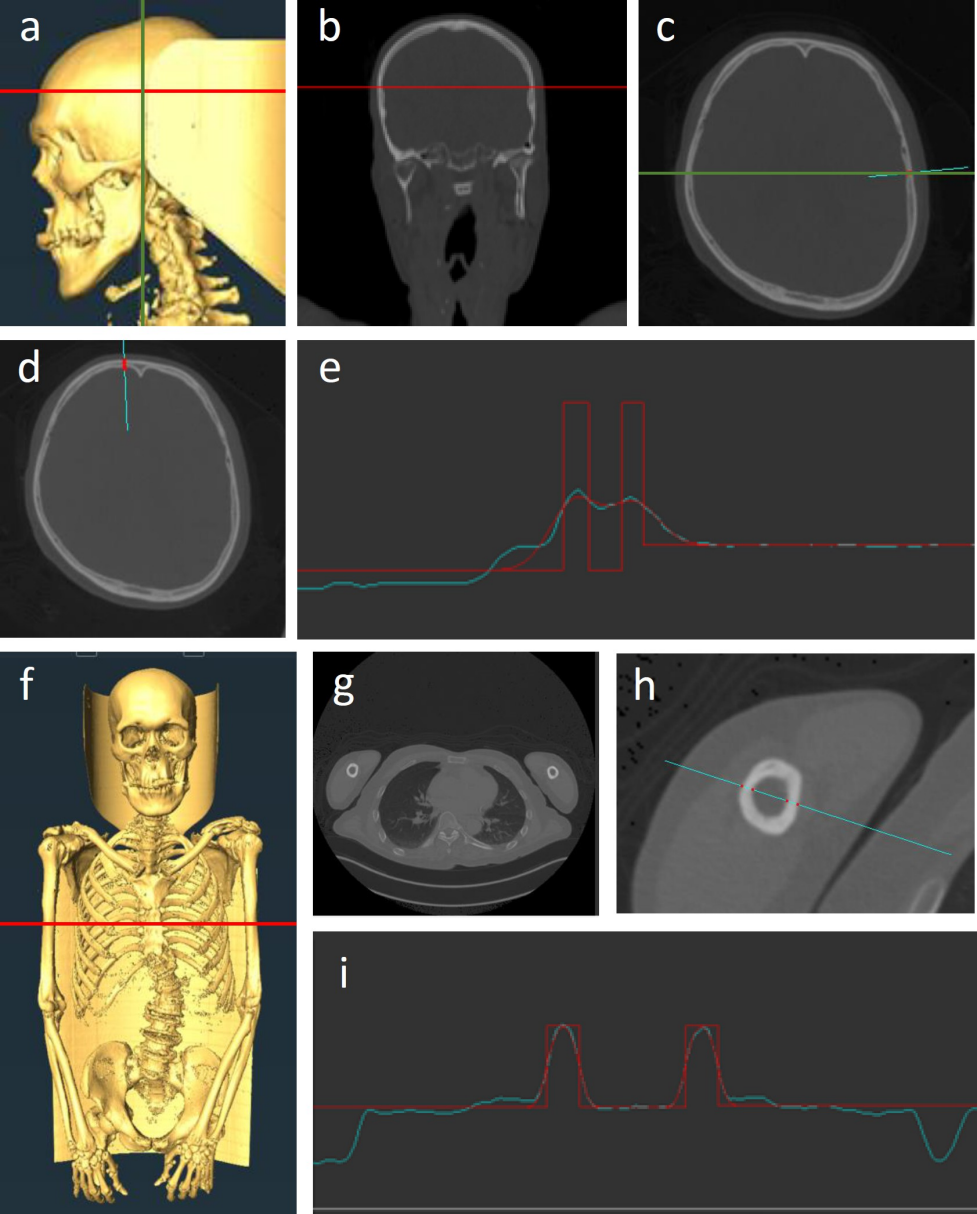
Measurement of bone thickness based on CT images. Measurement of TCVT (a-c), FCVT_full_, FCVT_cortical_ (d, e), HCT, and HBW (f-i) using Stradwin 5.4. (a-c) The green line denotes the coronal plane passing through the mandibular fossa. The red line denotes the transverse plane passing through the thinnest point of the temporal bone. (c, d, h) Measurement of cortical bone thickness along a line running through the cortex (the blue line). (e, i) Measurement is based on the intensity.
